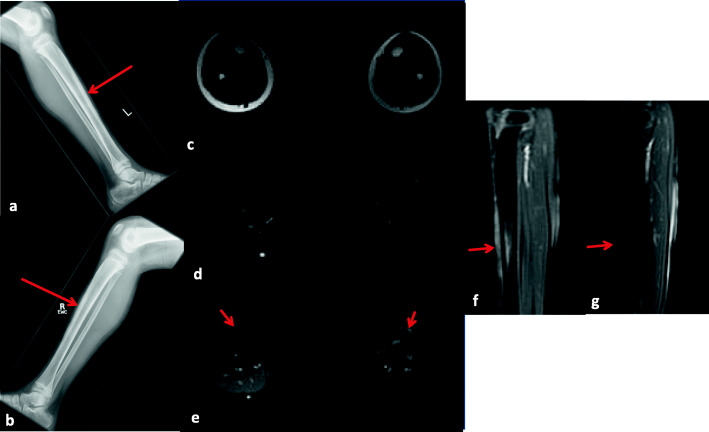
Case 3 Lateral radiographs of the left (a) and right (b) lower extremities (a, b) show prominence of the anterior mid cortex of bilateral tibias without associated periosteal reaction or bone abnormalities (arrows) Axial T1 (c), fat saturated T2 (d) and post-gadolinium T1 (e) MR images, and sagittal post-gadolinium T1 (f, left; g, right) MR images with the lower extremities were obtained 2.5 months after the aforementioned x-rays. These images show bilateral anterior periosteal thickening, increased T2 signal intensity and enhancement noted within the bone marrow and anterior soft tissues of the mid third of both tibias (arrows), more prominent on the left than on the right
